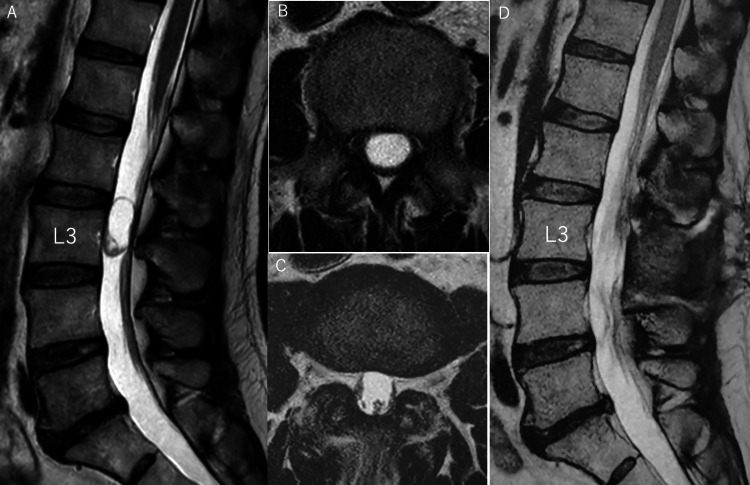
Pre- and postoperative MRI of case 1 (A) Preoperative MRI T2-weighted sagittal section showed the tumor including cysts from the L2/3 disc to the L3 vertebral body. (B) Preoperative MRI T2-weighted cross-sectional image showed that the tumor occupied the entire dural canal. (C) Postoperative MRI T2-weighted cross-sectional image showed no residual tumor. (D) Postoperative MRI T2-weighted sagittal section showed no residual tumor, and the L3 lamina had been removed.
